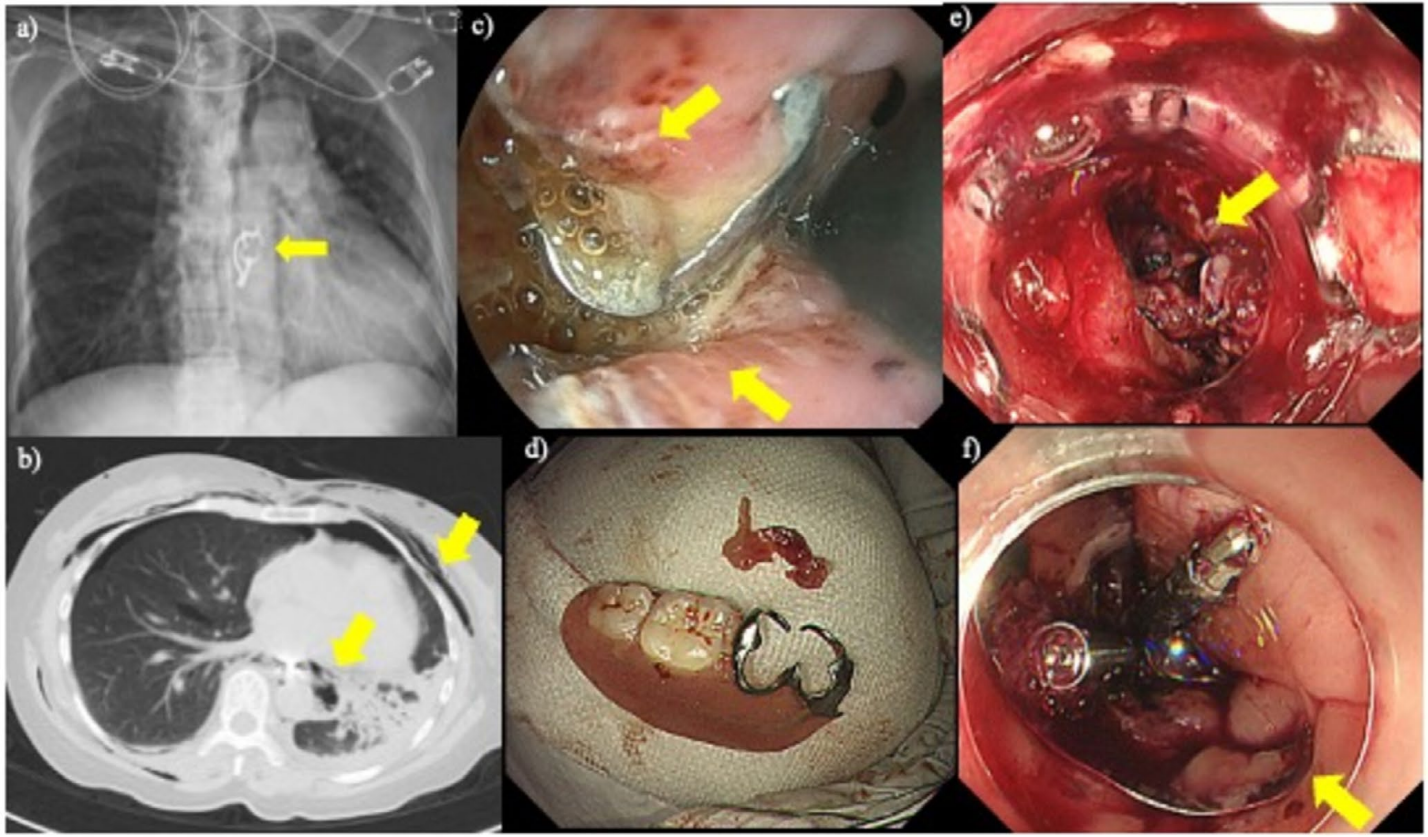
Endoscopic removal of esophageal foreign body (denture). (a) Esophageal foreign body (denture) on plain chest radiography (arrow). (b) Free air in the mediastinum on chest computed tomography (arrow). (c) Perforation of the middle esophagus caused by the foreign body (arrow). (d) Foreign body (denture) after removal. (e) The over‐the‐scope clip system was attached and perforation confirmed (arrow). (f) The endoscopic closure was successful (arrow).
